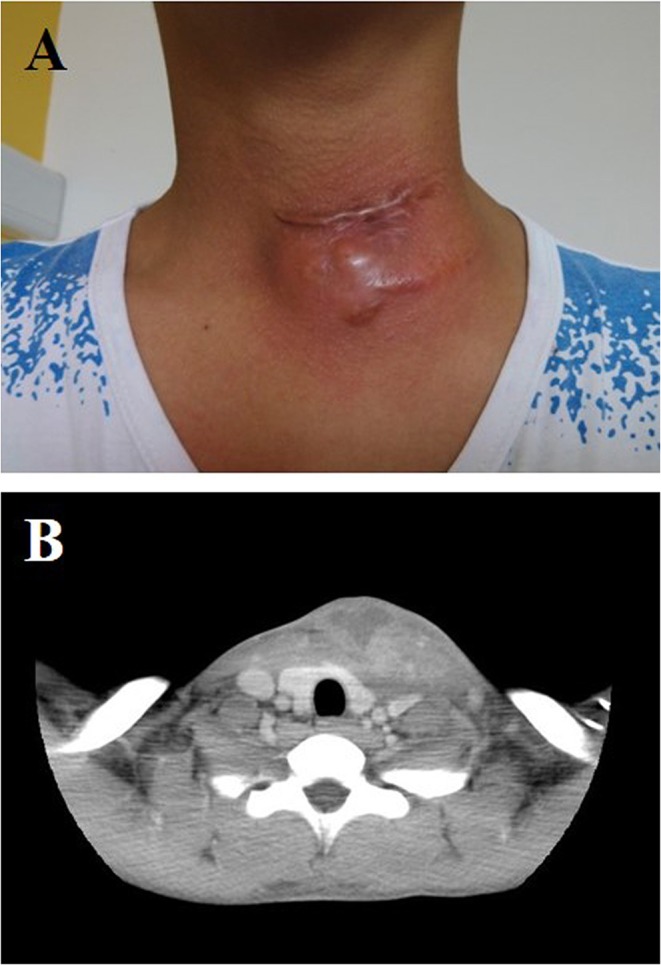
(A) Neck abscess formed 2 years after the primary operation in an 11-years-old boy (written informed consent was obtained from the patient and his parent for the publication of this image). (B) CT axial scan showed abscess in the left side of the neck.
